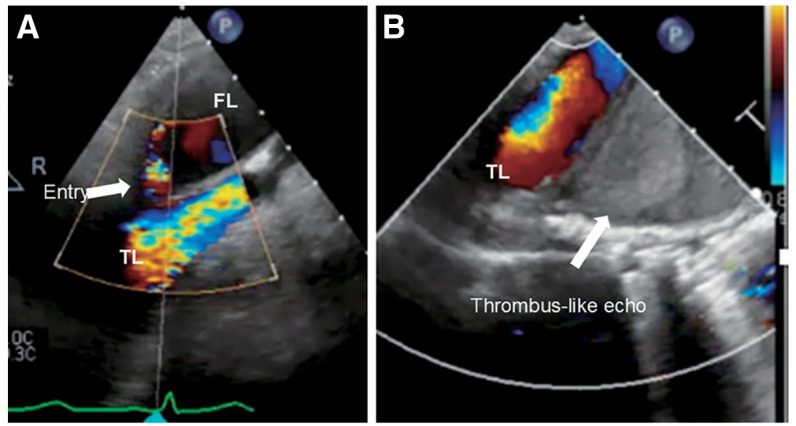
TEE findings. (A) Color Doppler imaging demonstrated an entry tear in the descending thoracic aorta, with flow communication from the TL to the FL. (B) When the FL was traced proximally toward the aortic arch, flow became markedly stagnant, with spontaneous echo contrast and thrombus-like echogenic material. TL, true lumen; FL, false lumen; TEE, transesophageal echocardiography
